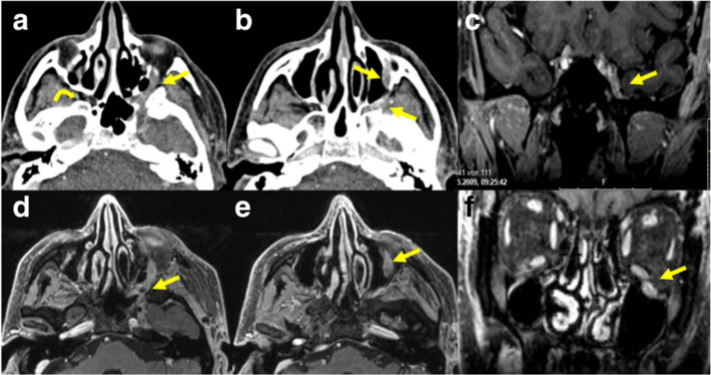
Patient with adenoid cystic carcinoma and perineural extension along the inferior orbital nerve, the pteryopalatine fossa and the maxillary nerve on the left side. Contrast enhanced CT images a, b showing the asymmetric thickened enhancing nerve (arrows) on the left. The curved arrow a shows the normal fat density of the pterygopalatine fossa on the right side. Axial T1-weighted images d, e showing the thickened enhancing nerve (arrows) on the left. Coronal T1-weighted images with fat saturation after injection of gadolinium c showing asymmetrical nerve enhancement (arrow) in the cavernous sinus and f the thickened infraorbital nerve on the left (arrow)
